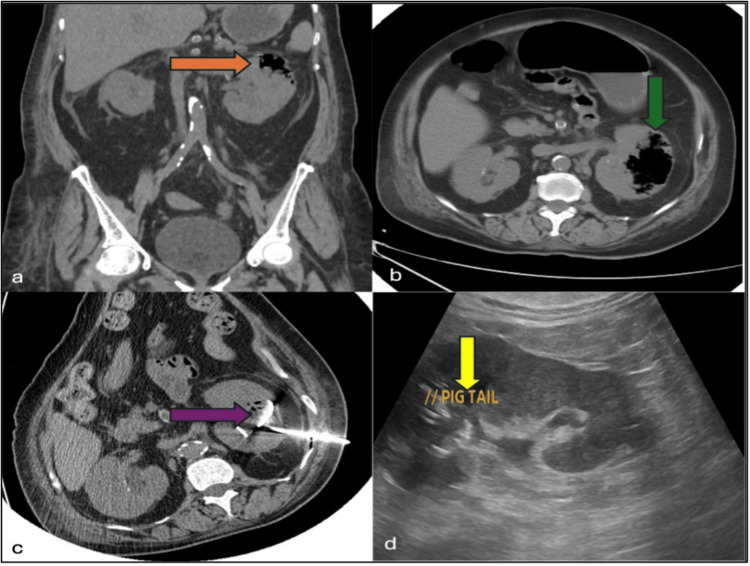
Multimodality Imaging of Emphysematous Pyelonephritis Demonstrating Gas-Forming Infection and Percutaneous Drainage. (a, b) Coronal and axial CT imaging showing the gas in the renal parenchyma. (c, d) CT and Ultrasonogram images depicting the placement of a percutaneous nephrostomy.
